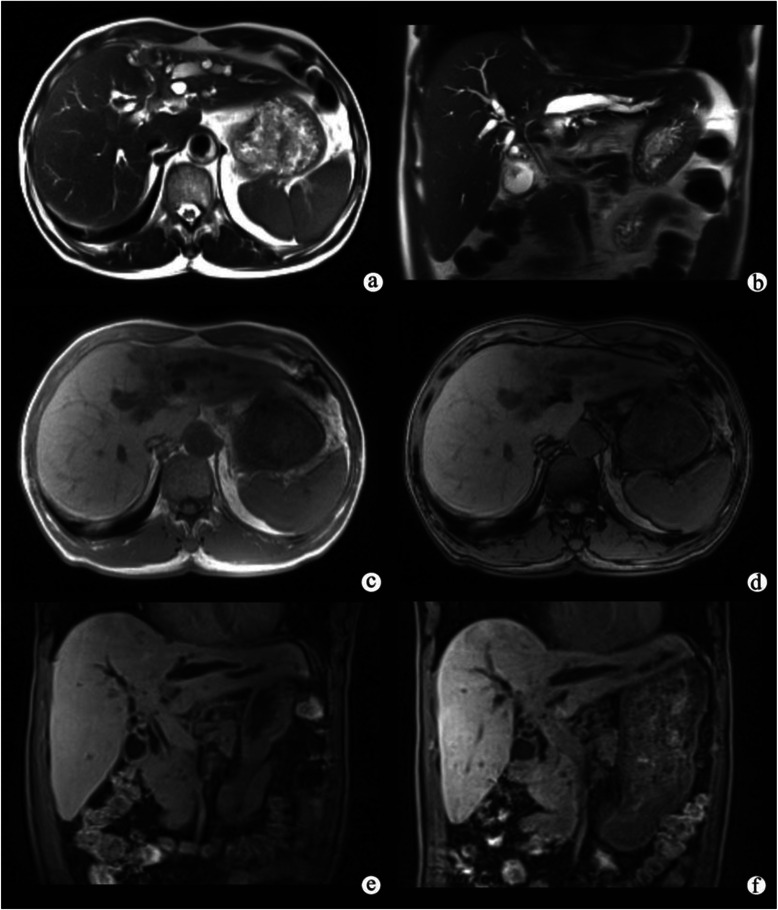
Infiltrative hilar cholangiocarcinoma in a patient with progressive jaundice. A axial (a) and coronal (b) T2-weighted image showed wall thickening of bile duct at the hepatic hilar level, and the left hepatic ducts were dilated. In (c) and out (d) of phase imagings showed no change on the signal intense of liver background. The hepatocellular phase (e) showed nearly no Gd-EOB-DTPA in the common bile duct. The biliary phase (f) showed that there was little Gd-EOB-DTPA in the common bile duct, indicating that the function of biliary system was abnormal.
